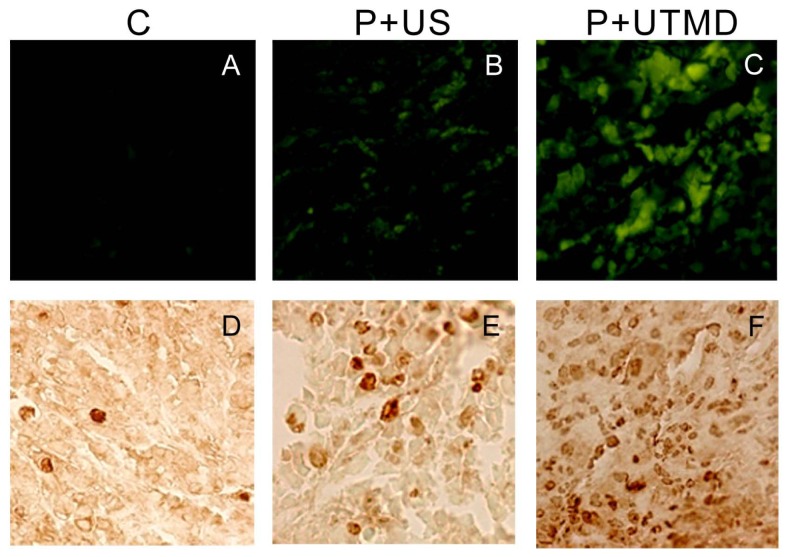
Detection of TUNEL expressions in different groups (400×). C: control group; P + US: plasmid + ultrasound irradiation group; P + UTMD: plasmid + microbubble + ultrasound irradiation group, A–C: Tunel assay; D–F: DAB detection.
